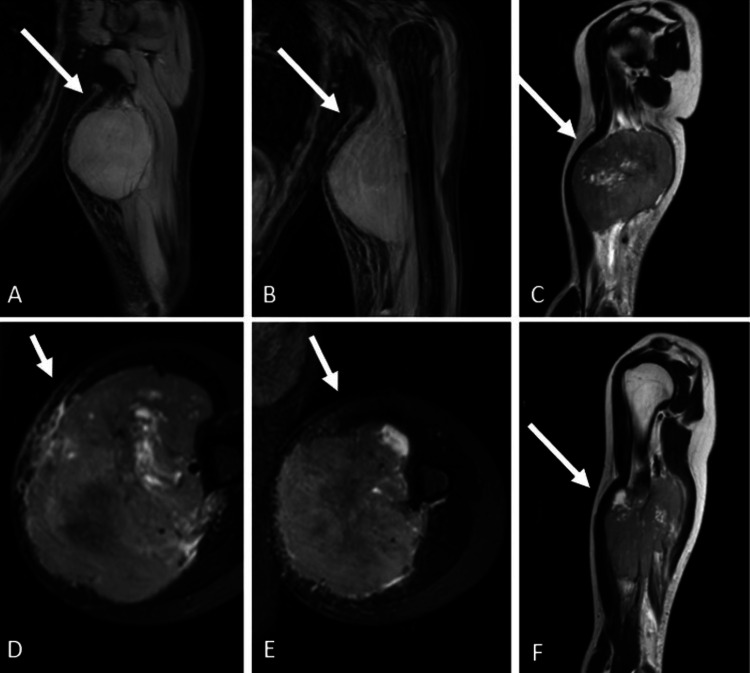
Preoperative magnetic resonance imaging (MRI) of the second patient, an 18-year-old female, showing the lesion located in the left shoulder region, surrounding the biceps brachii muscle: (A,B,C,F) sagittal and (D,E) axial MRI sections. The white arrows indicate the lesion surrounding the biceps, triceps, and brachioradialis muscles.
